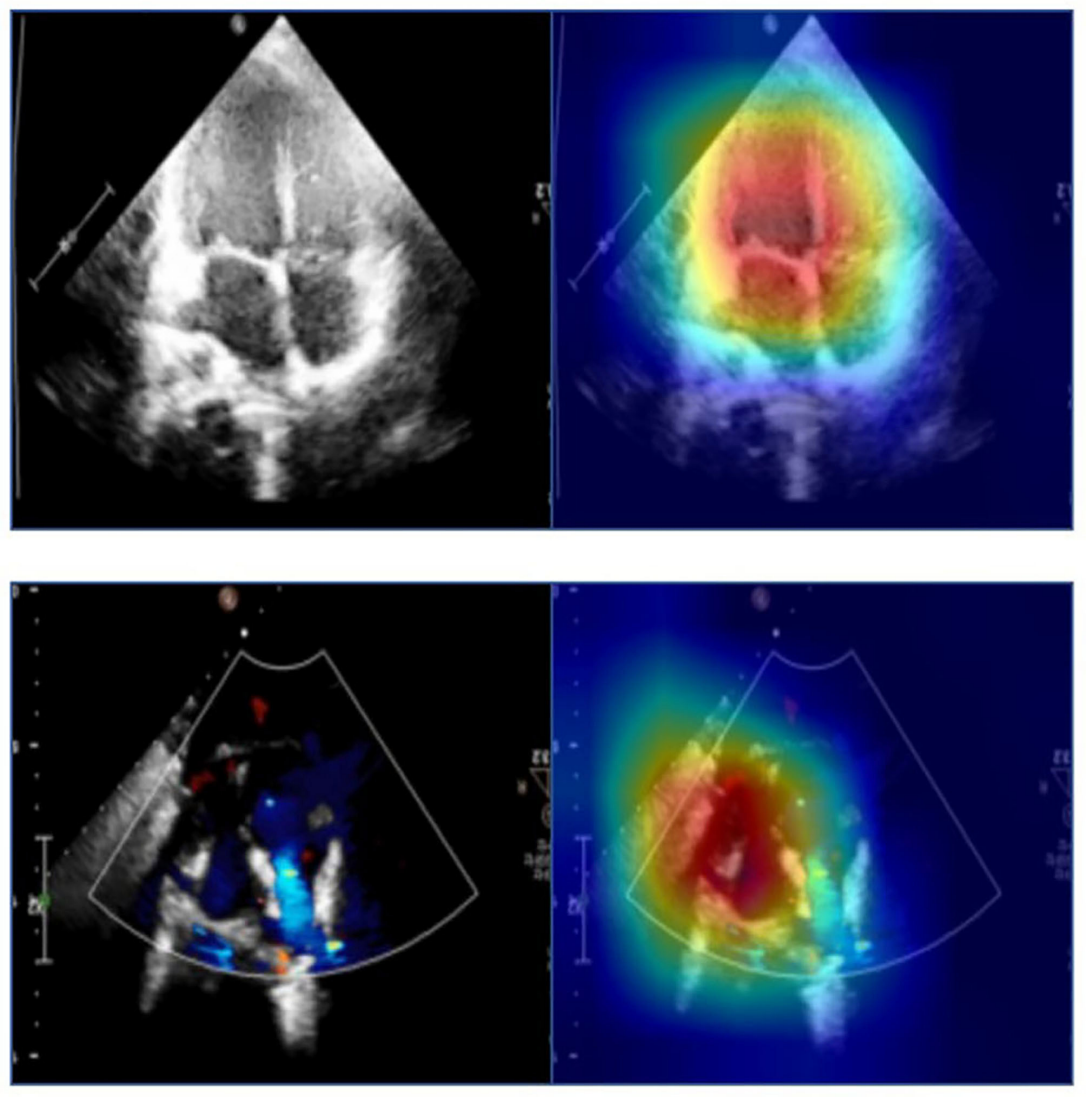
The activation maps of the apical four-chamber view and the subcostal sagittal view of the atrium septum. Different colors in the activation map represent different weights in model prediction. The red part has a higher weight and the blue part has a lower weight.
